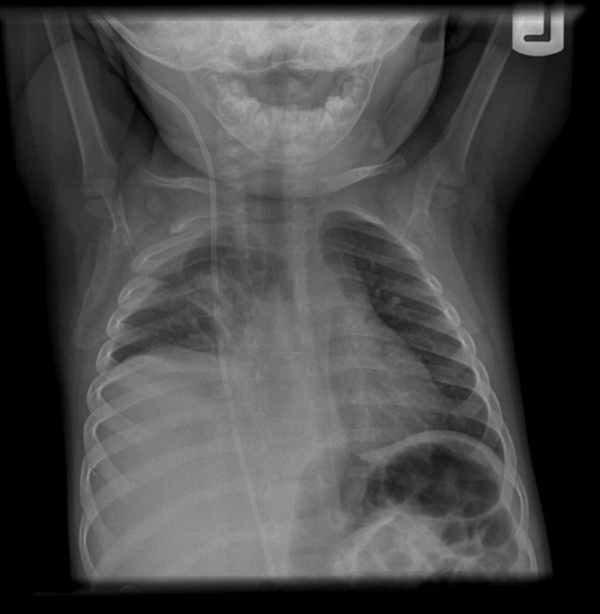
Anteroposterior radiograph demonstrating a moderate to large right-sided pleural fluid collection which is predominantly subpulmonic in location.
